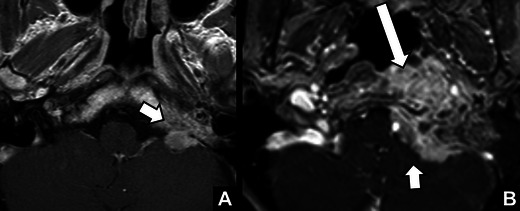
Skull base metastasis.*A 66-year-old man with a history of oropharynx carcinoma treated with radiation therapy 1 year earlier presenting with left VCP. FDG-PET revealed a hypermetabolic lesion in the left skull base presumed to be a metastasis. a Axial T1-weighted contrast-enhanced images at the level of the skull base showed a heterogeneously enhancing mass in the left cerebellomedullary cistern (*arrow*). The mass shows infiltrative growth into the skull base and jugular foramen, and thereby the vagal nerve, causing left VCP. The imaging findings were suspicious for metastatic disease. b Follow-up T1-weighted contrast-enhanced images with fat saturation showed progression of the skull-base mass with infiltrative growth into the nasopharynx (*long arrow*) and mass effect on the cerebellum and pons (*short arrow*)
